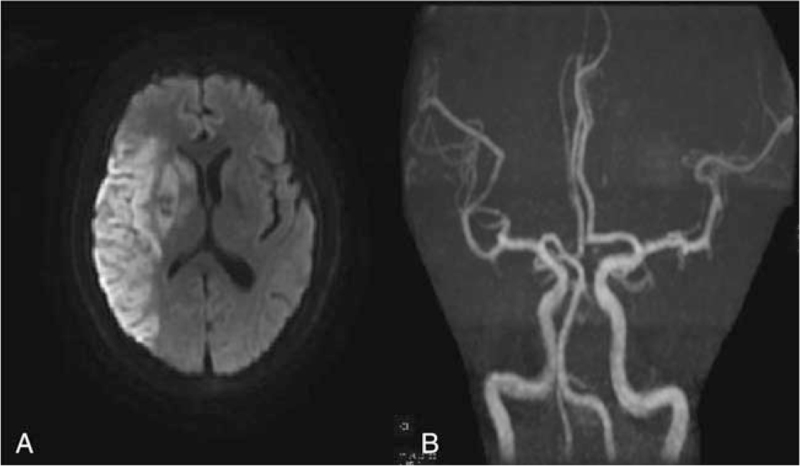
(A) Brain magnetic resonance imaging showed that the right frontal lobe, temporal lobe, occipital lobe, parietal lobe, insular lobe, radial crown and basal ganglia had massive acute cerebral infarction, and the right basal ganglia had small hemorrhage after infarction. (B) The right internal carotid artery was unobstructed.
